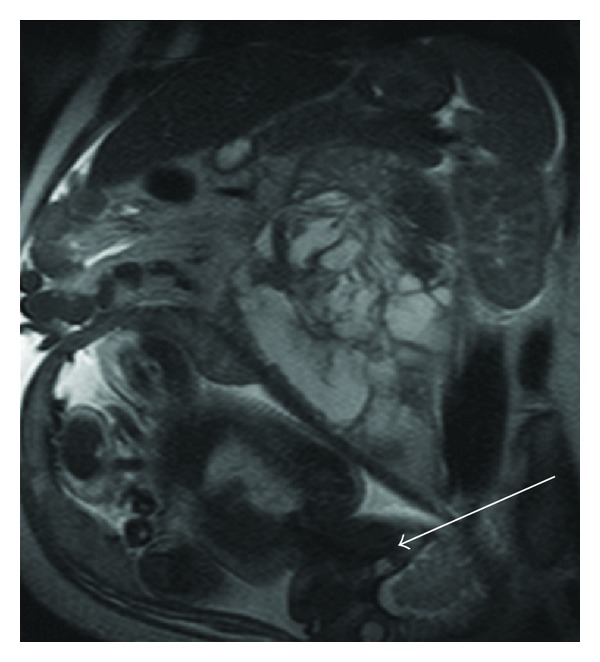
MRI abdomen, arrow pointing to gravid uterus.
